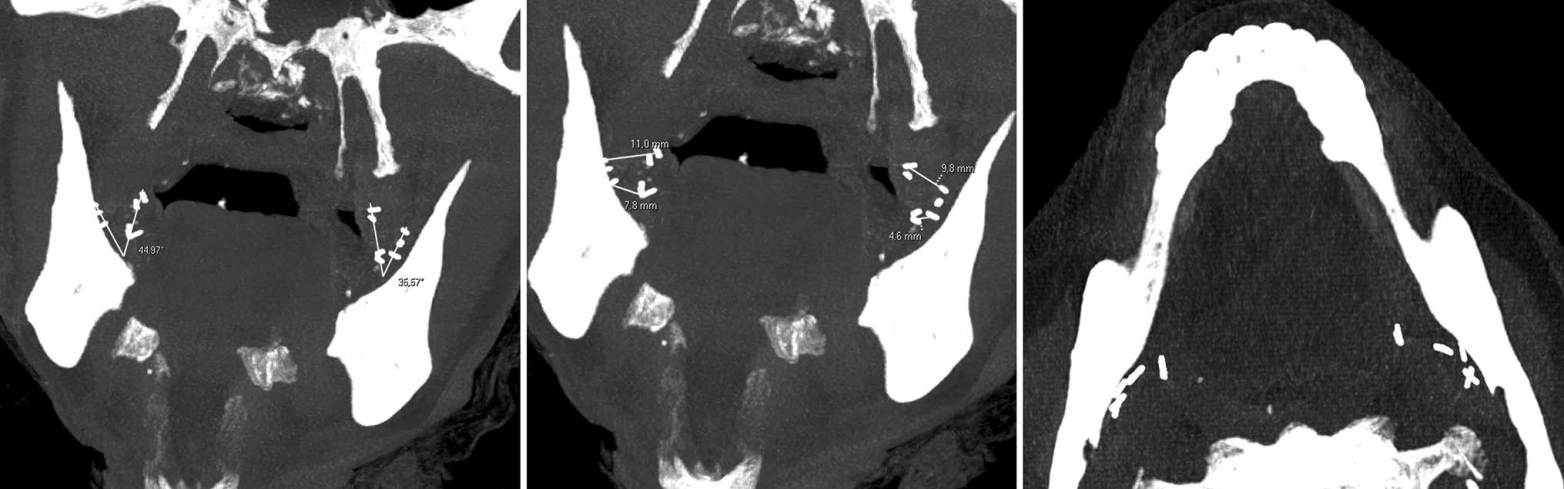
Coronal and axial CT maximum intensity projection reconstruction shows how measurements were performed on a patient
